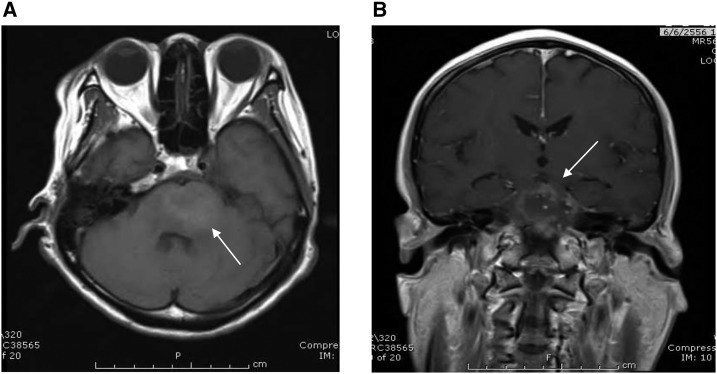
Magnetic resonance imaging of brain showed a large heterogeneous lesion and perilesional edema on the T1-weighted image (A) with rim enhancement (B) at pons.
